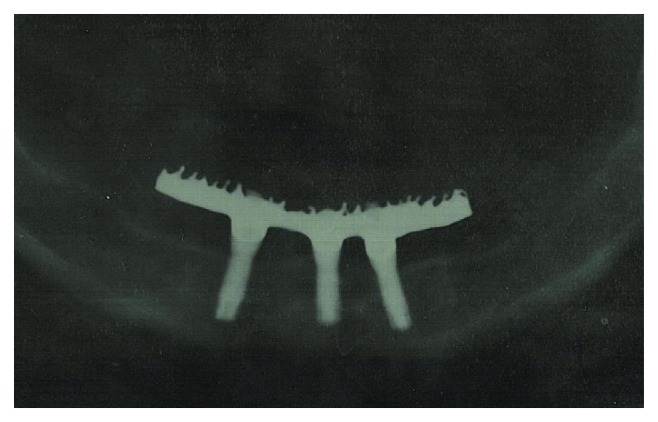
One-year postoperative follow-up panoramic radiograph.
